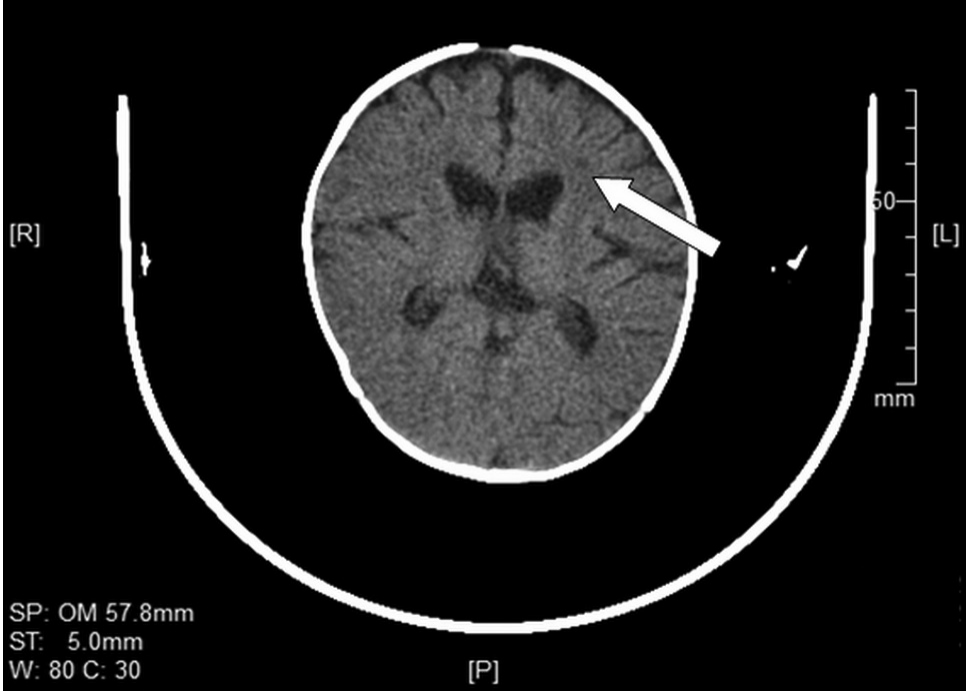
Computed tomography scan of the brain showing microlacunar hypodense zones in periventricular white matter of the frontal lobes.
